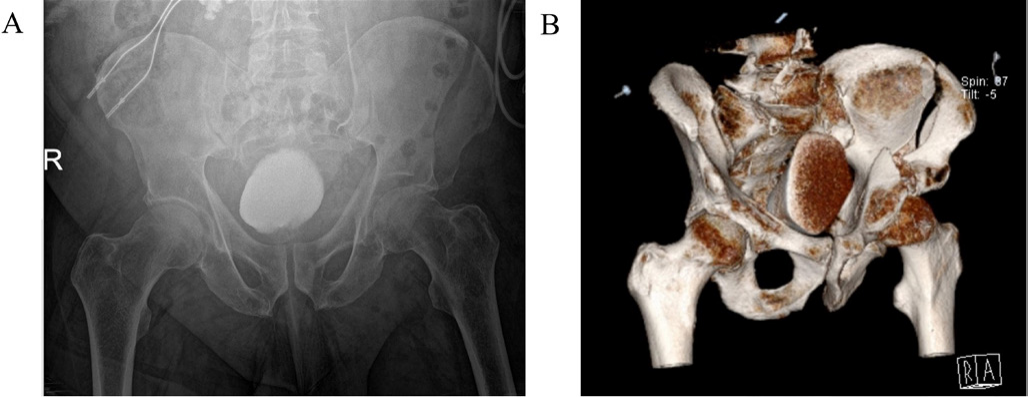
Radiological investigations for acetabular fracture. (A) Plain film radiograph demonstrating left-sided acetabular fracture, involving the anterior and superior columns. (B) Three-dimensional reconstruction of CT of the pelvis demonstrating a moderately displaced fracture of the left iliac bone, which extends from the left acetabulum, where there is a comminuted intra-articular fracture involving the acetabular root, anterior and posterior columns of the acetabulum and left proximal superior ramus.
